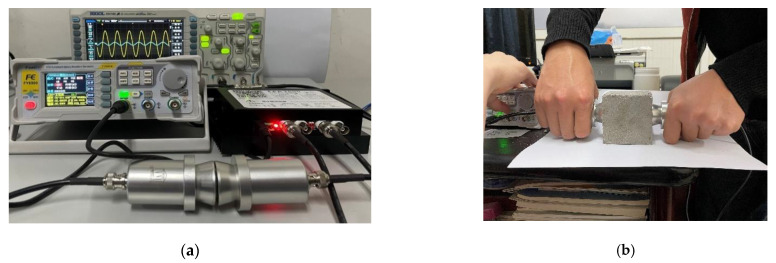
(a) Ultrasonic test instruments; (b) ultrasonic test.
